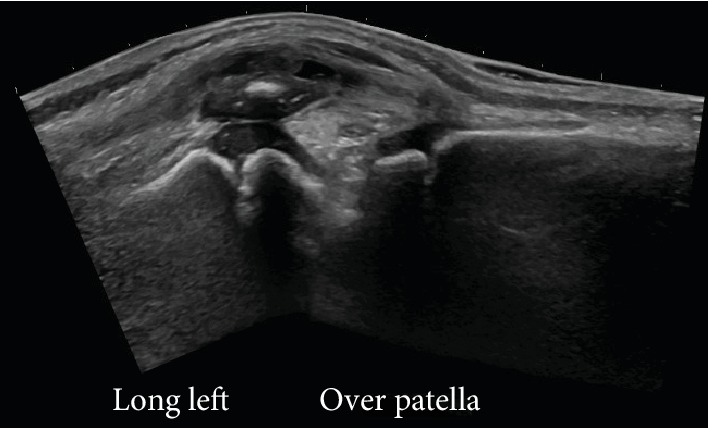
Ultrasound of the affected knee confirms a collection of fluid within the prepatellar bursa.
